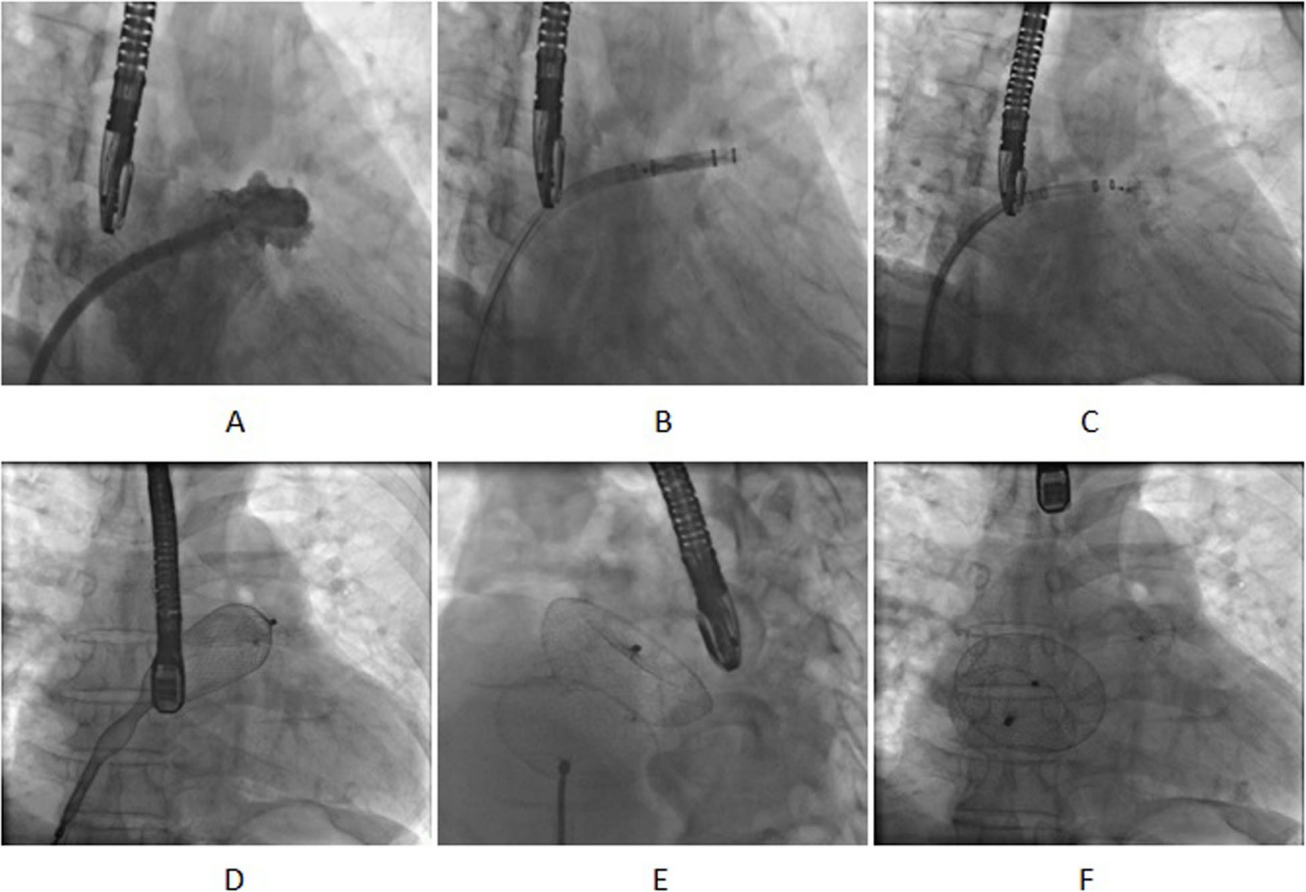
X-ray imaging of the process of LAAC and ASD occlusion (LAA angiography(a), LAA occluder were sent into the LAA(b), A pull test was conducted to judge the stability of the LAA occluder(c), ASD/PFO plug devices were sent into left atrium(d), A pull test was conducted to judge the stability of the ASD occluder(e), the LAA and ASD occluder were released(f)
